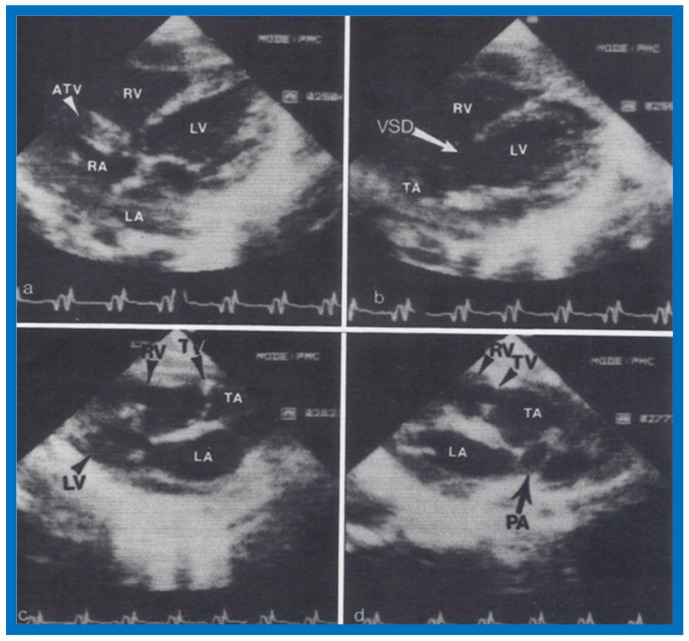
Two-dimensional echocardiographic video frames demonstrating (a) atretic tricuspid valve (ATV) between the right atrium (RA) and right ventricle (RV), (b) a large subtruncal ventricular septal defect (VSD), (c) thickened and somewhat domed truncal valve (TV) leaflets, and (d) origin of the pulmonary artery (PA) from the posterior aspect of the truncus arteriosus (TA). LA, Left atrium; LV, left ventricle. Reproduced from Rao P.S., et al. [22].
